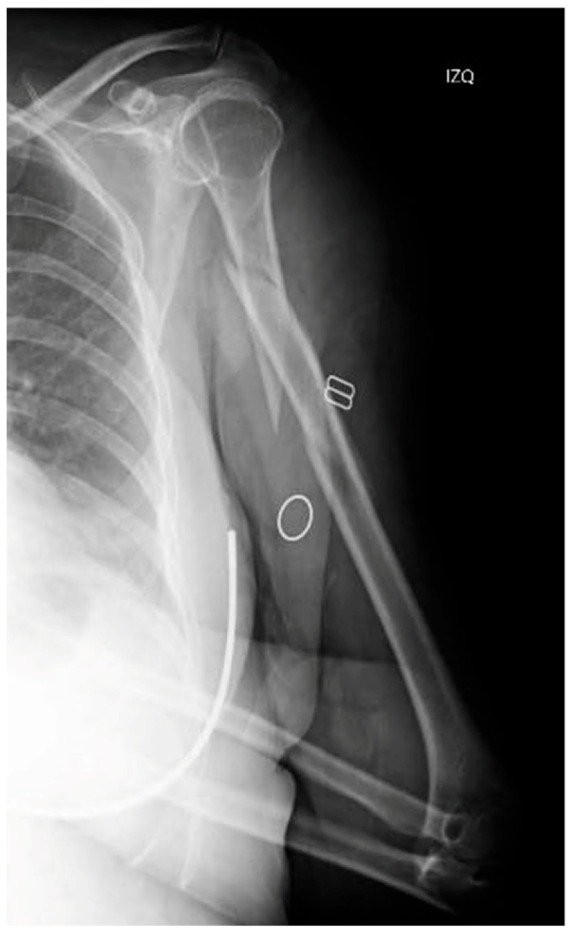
X-ray of the initial injury, showing a proximal humerus fracture extending into the diaphysis.
